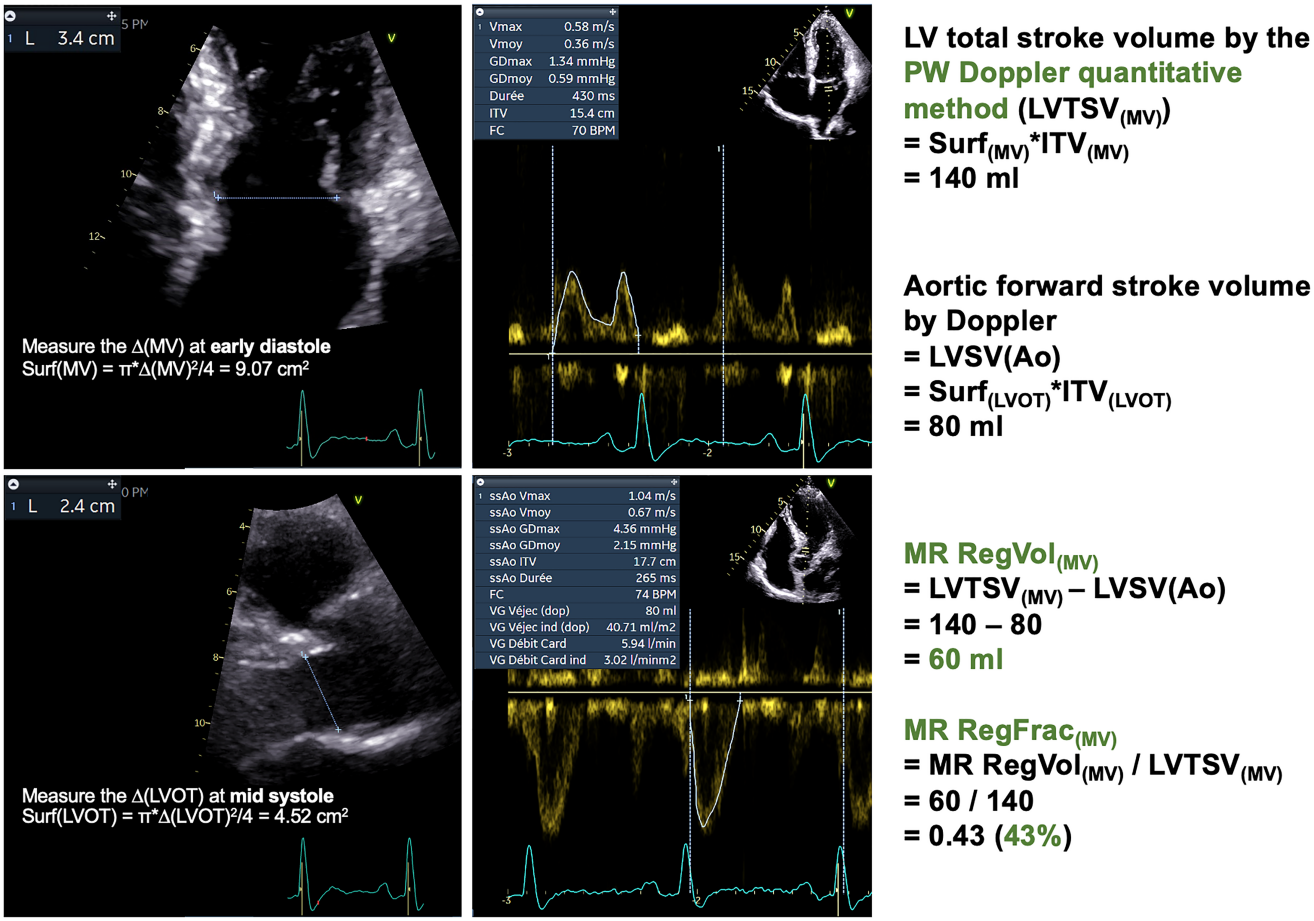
PW Doppler quantitative method. Same patient as Figure 5. The LV total stroke volume is computed by the Doppler method as follows: LVTSV_(MV)_ = π x (Δ_MV_)2 x VTI_MV_/4. Importantly, the *Δ*_MV_ must be measured at early diastole. Also the sample volume must be positioned at the level of the mitral annulus (not that of the leaflet tips). The aortic systolic forward stroke volume is computed by the Doppler method as follows: LVSV(Ao) = π x (Δ_LVOT_)2 x VTI_(LVOT)_/4. The *Δ*_LVOT_ must be measured at mid systole. The MR RegVol_(MV)_ is computed as the difference between the LVTSV_(MV)_ and the LVSV(Ao). Consequently, the MR RegFrac_(MV)_ is obtained by dividing the MR RegVol_(MV)_ by the LVTSV_(MV)_. *Δ*, diameter; LV, left ventricular/ventricle; LVOT, LV outflow tract; LVSV(Ao), LV systolic aortic forward stroke volume by Doppler method; LVTSV(_MV_), LV total stroke volume by the PW Doppler quantitative method; MR, mitral regurgitation; RegVol, regurgitant volume; RegFrac, regurgitant fraction; PW, Pulsed-Wave; VTI, velocity-time integral.
